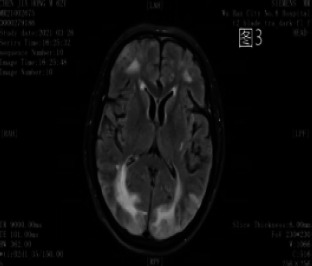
Multiple punctate and patchy hyper-intensities in the left basal ganglia and bilateral frontal and parietal lobes on FLAIR
